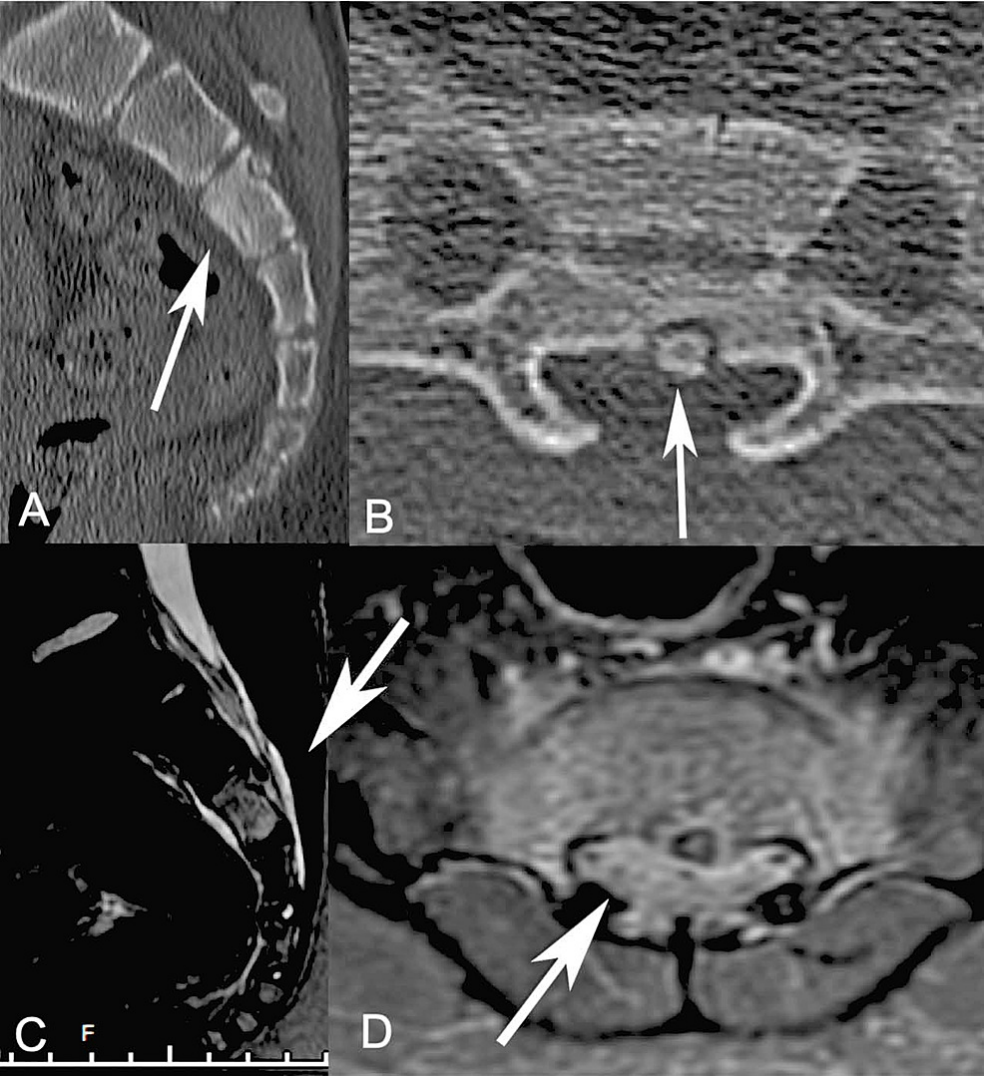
Pre-operative imaging: lumbo-sacral CT scan (A, B) and MRI (C, D) showing an osseous lesion originating from the posterior S3 body
